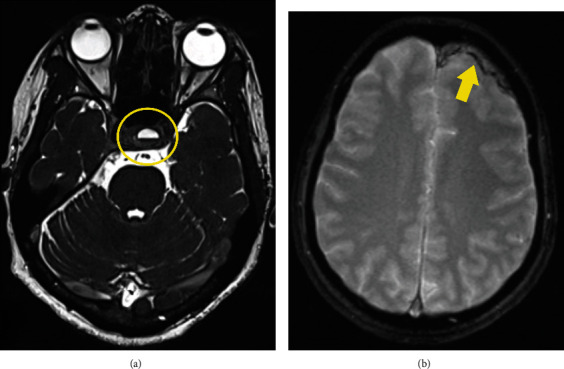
(a) CISS 3D MRI image shows the level of fluid debris in the pituitary fossa (circle). (b) T2∗-weighted gradient-echo MRI image shows the signal intensity of a subdural hematoma (arrow).
